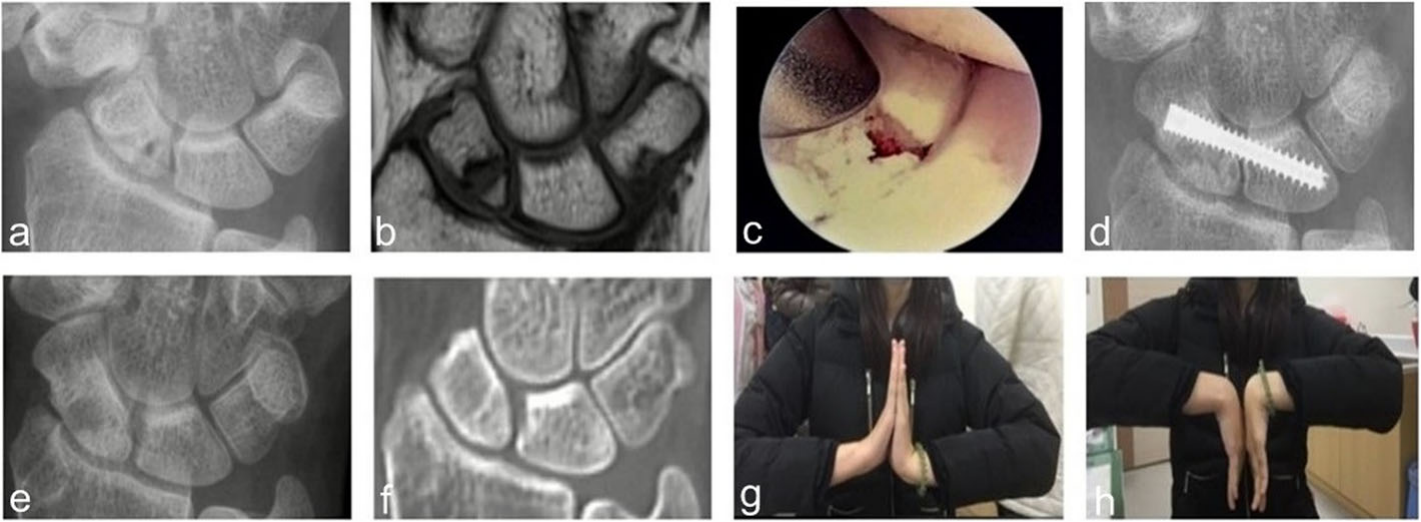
A 19-year-old female. (Case 18) (a) and (b) Radiograph and MRI 9 months after the fracture event showing the right scaphoid proximal pole nonunion. (c) Arthroscopic bone grafting. (d) Radiographs at the postoperative 1-month follow-up. (e) and (f) Radiograph and CT at postoperative 40 months, revealing a solid union. (The screw was removed 6 months after surgery). (g) and (h) The patient regained nearly full range of motion of the wrist
